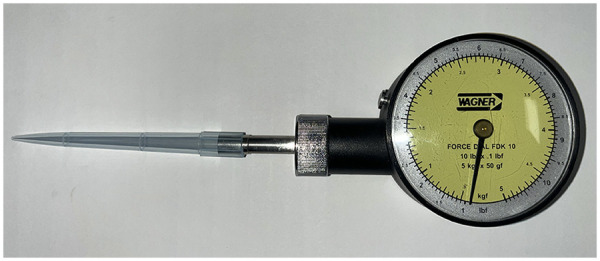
The modified algometer used in this study. A handheld algometer (Wagner Force Dial^TM^ FDK Series Push Pull Force Gage, FDK 10; Wagner Instruments, CT, USA, Maximum force 5 kgf) with a modified 1,000 µL pipette tip (Thermo Fisher Scientific) which was cut approximately 20 mm from the base and firmly attached to the Push gauge.
